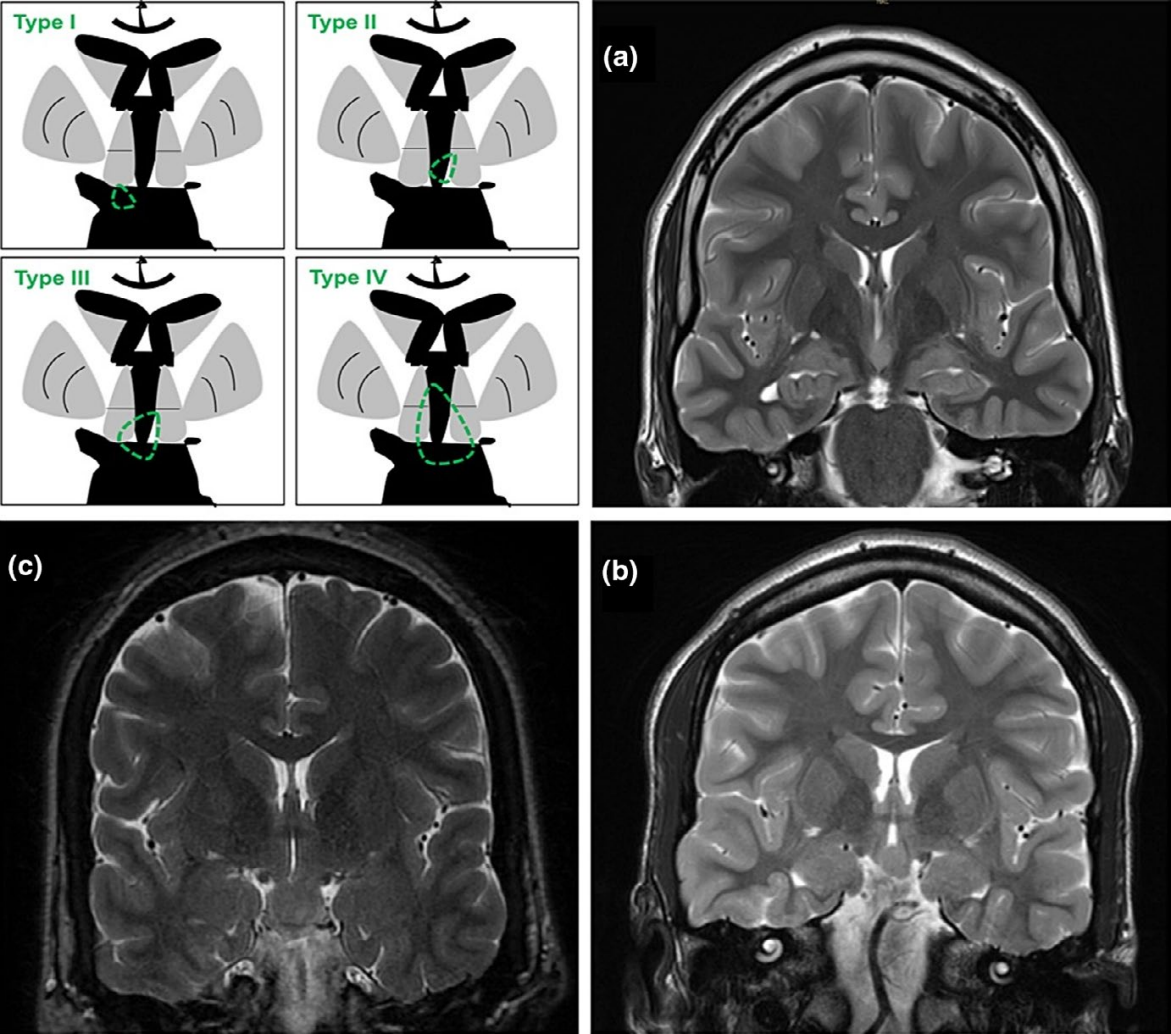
Neuroimage. (a) MRI slice in the coronal plane using T2 weighted image from patient No. 2. An intrahypothalamic hamartoma lesion is attached to the left lateral wall of the third ventricle and completely above the floor of the third ventricle (type II). This patient also had left side hippocampal sclerosis (dual pathology). (b) Same sequence belonging to patient No. 8. There is a HH arising from the left wall of the third ventricle that extends beyond its inferior limit (Type III). (c) Patient No. 5 with a giant lesion emerging from the inferior walls of the third ventricle (Type IV)
